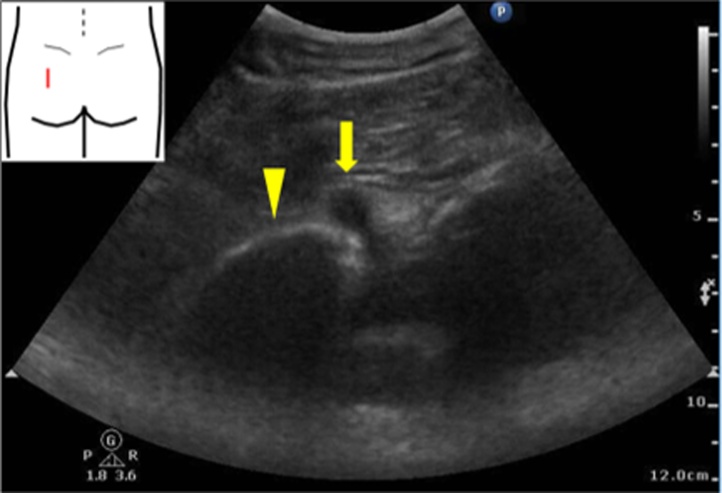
Ultrasonographic imaging shows an invaginated left ureter (arrow) and the ilium (arrowhead) when the probe was placed on the left buttock.
